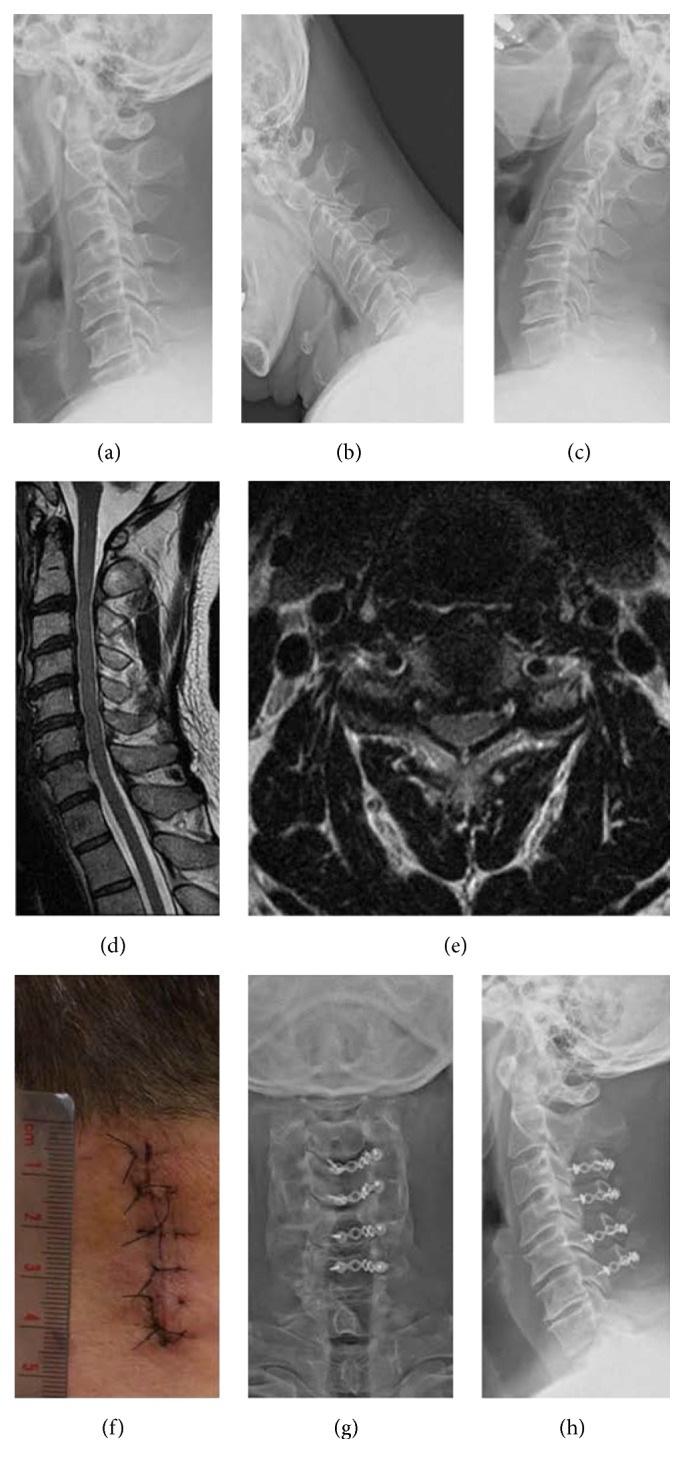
Preoperative X-ray in this case showed C3–C7 spondylosis (a) without segmental instability and local kyphotic deformity (b and c). T2 weighted MRI revealed C3–C7 stenosis at sagittal plane (d) and banana shape of the compressed spinal cord at axial plane (e). The surgical wound was about 4 cm (f). Postoperative plain films showed well alignment of C3–C6 laminoplasty and C7 partial laminectomy at anterior to posterior (g) and lateral (h) views at 1 month.
